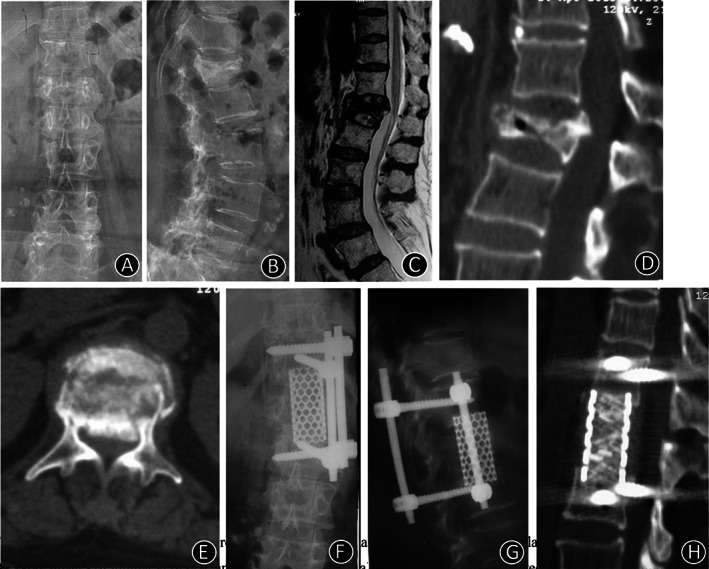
Clinical imaging from one representative patient (62‐year‐old woman) who complained of lumbar 1 compression fracture. (A, B) X‐ray images of lumbar vertebrae anteroposterior and lateral position X‐ray films showed L1 vertebral compression fracture. (C) T2‐weighted sagittal preoperative MRI of the lumbar spine showed that the spinal cord was compressed by a bone fragment. (D, E) The lumbar vertebrae CT indicates that the bone fragment had burst into the vertebral canal. (F, G) At 12 months postoperatively, the X‐ray imaging indicated that the location of the internal fixator was excellent. (H) At 12 months postoperatively, two‐dimensional CT indicated that the auto graft bone fusion was excellent.
